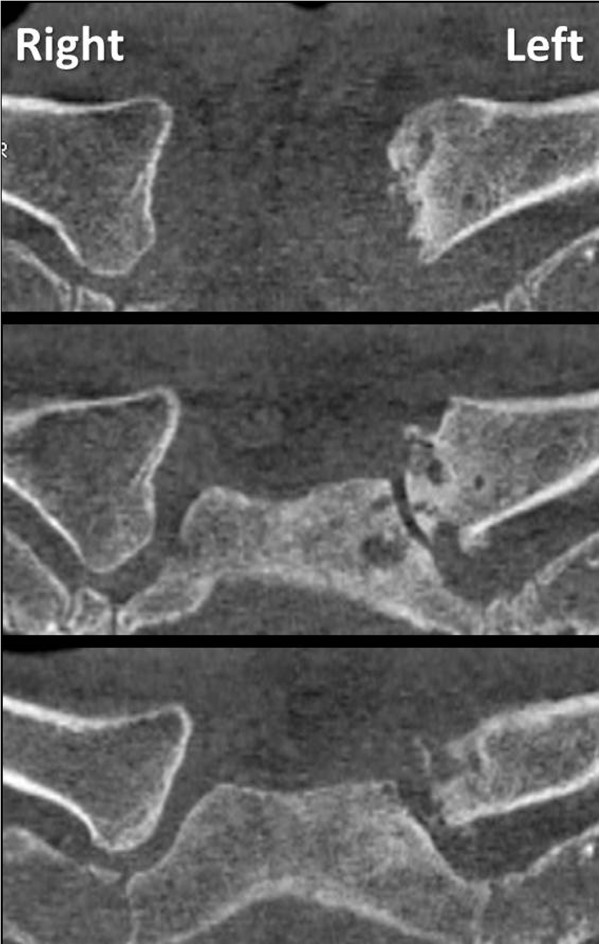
Follow-up CT scan at 9 months post injury (7 months after hardware removal) demonstrates early posttraumatic arthritis of the left sternoclavicular joint, compared to the contralateral (uninjured) right side.
